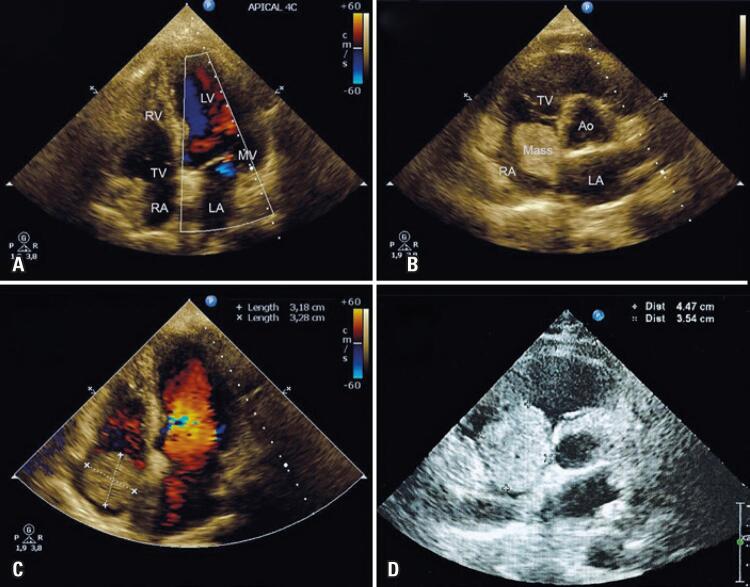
Echocardiography evolution of the right atrial mass. (A) Previous negative two-dimensional transthoracic echocardiogram showing apical four-chamber view and clear right atrium. (B and C) Diagnostic transthoracic echocardiogram (6 months later) showing aortic valve level (B) and apical four-chamber view (C), with a large homogenous right atrial mass attached on the atrial septum, measuring 3.18x3.28cm. (D) Pre-operative two-dimension transesophageal echocardiogram in mild-esophageal four-chamber view (6 months after diagnosis) demonstrating the rapidly growth of the right atrial mass, remaining attached on atrial septum and measuring 4.47x3.54cm
